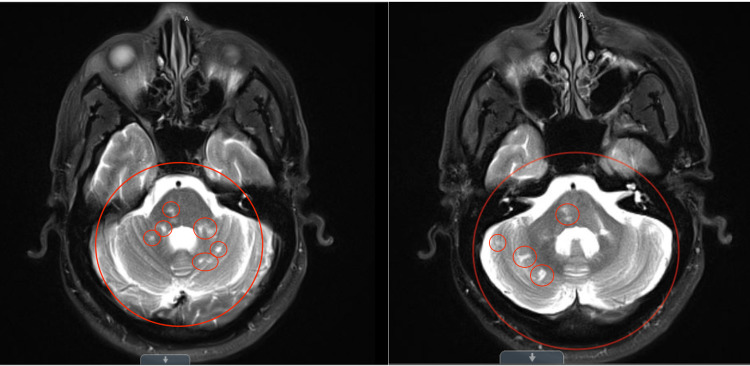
Axial T2-weighted sequence demonstrating remote left middle cerebellar peduncle infarct, and border zone infarcts within the cerebellar hemisphere (circled).
